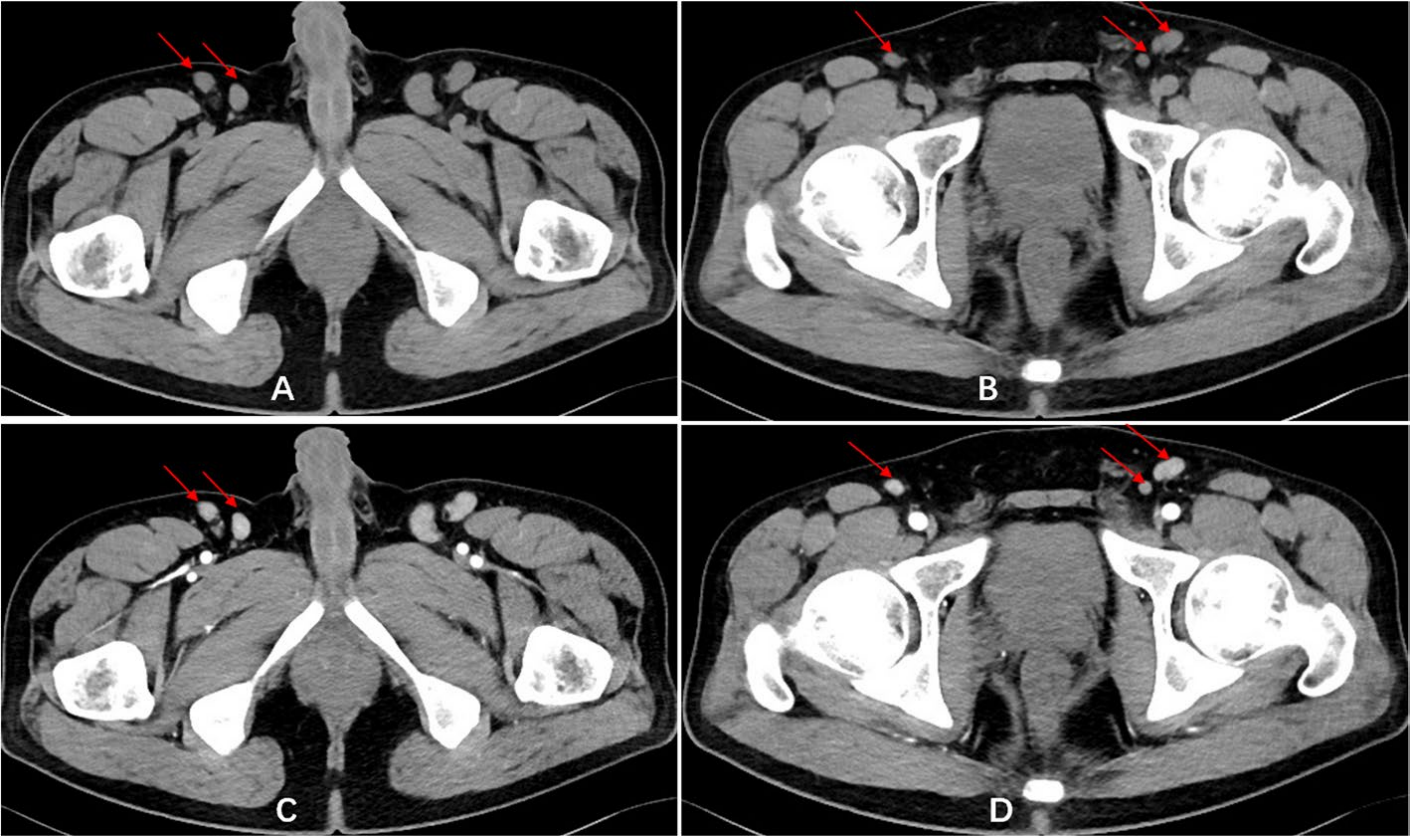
The lower abdomen CT. A and B reveal multiple nodular masses in the groin on both sides; C and D reveal inhomogeneous contrast enhancement in the arterial phase
